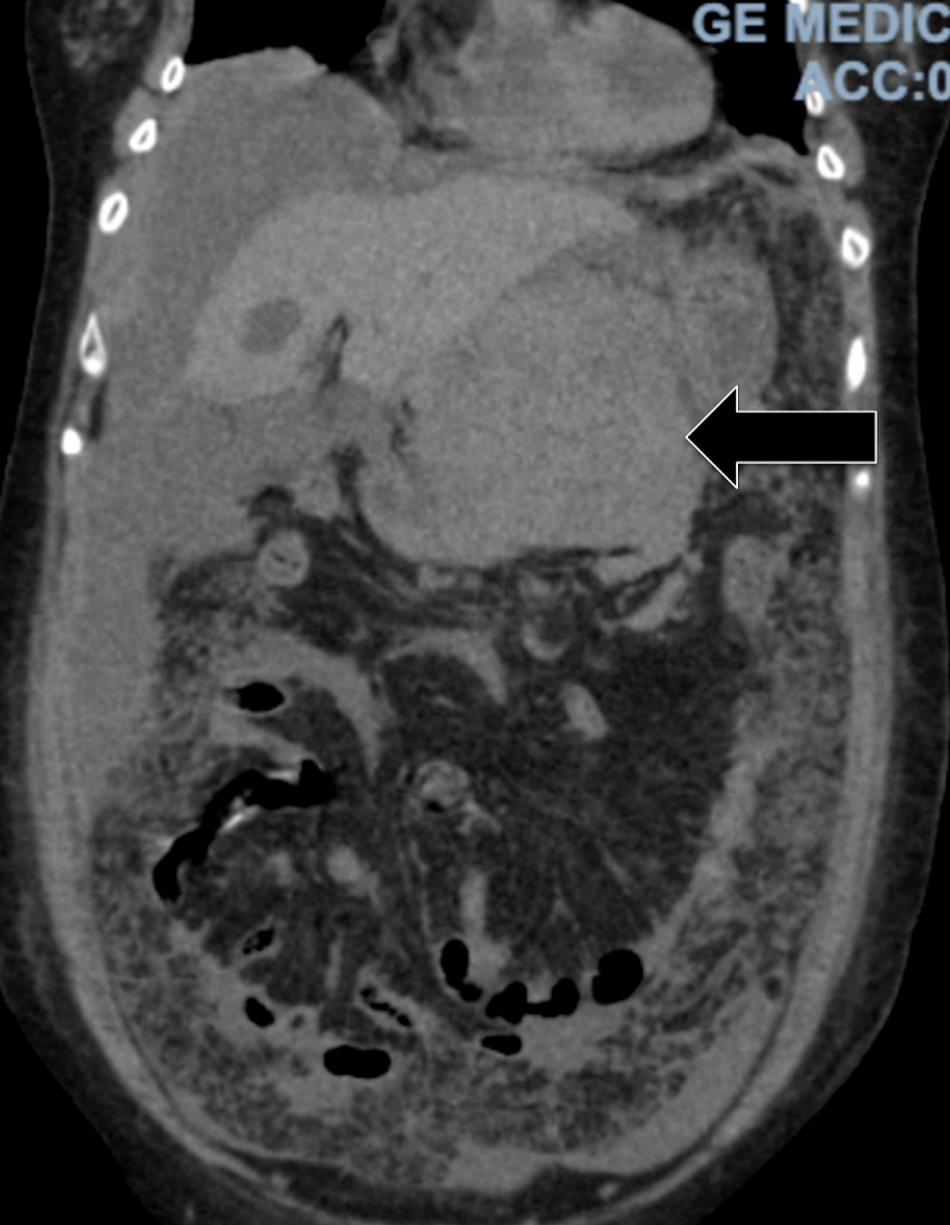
Repeat CT scan of the abdomen performed two weeks following admission The image shows an interval increase in the size of the mass extending from the stomach, as well as new metastatic liver lesions and moderate ascites (arrow) CT: computed tomography
